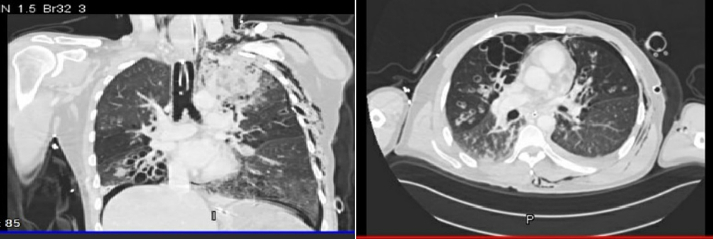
For case 2: CT scan shows pre-existing cystic bronchiectasis, extensive surgical emphysema on the left side of the chest extending to the neck and abdominal wall.
